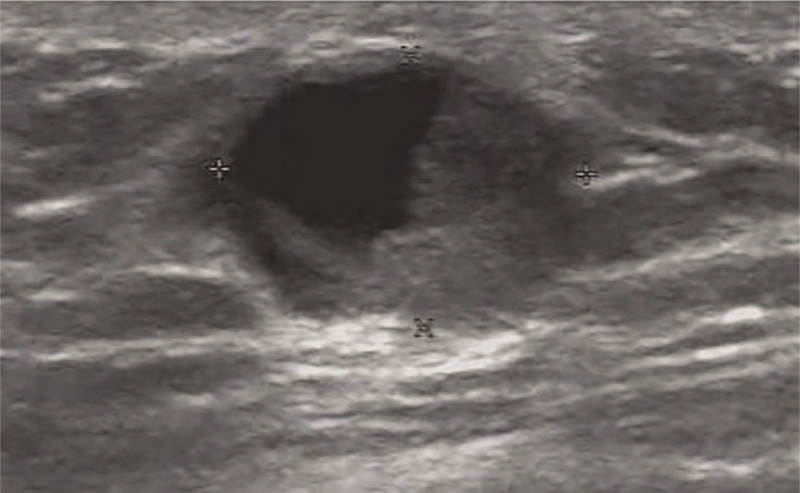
Ultrasound of the right breast demonstrated a well-circumscribed, complex mass.
